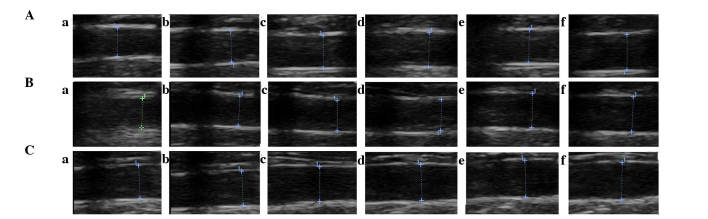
Treatment with ML7 improves the vascular endothelial function of experimental AS in rabbits. Vascular endothelial function was measured by transcutaneous noninvasive ultrasound. Longitudinal axis view of the abdominal aorta in B-mode ultrasound image. (A) Control group; (B) AS group; (C) ML7 group. Rabbits under sedation were serially infused for 2 min at 10-min intervals each in the marginal ear vein with (a) saline at 1 µg/ml/min, (b) Ach at 1.5 µg/ml/min, (c) Ach at 3 µg/ml/min, (d) Ach at 6 µg/ml/min, (e) NTG at 5 µg/ml/min and (f) NTG at 7.5 µg/ml/min. AS, atherosclerosis, Ach, acetylcholine; NTG, nitroglycerin.
